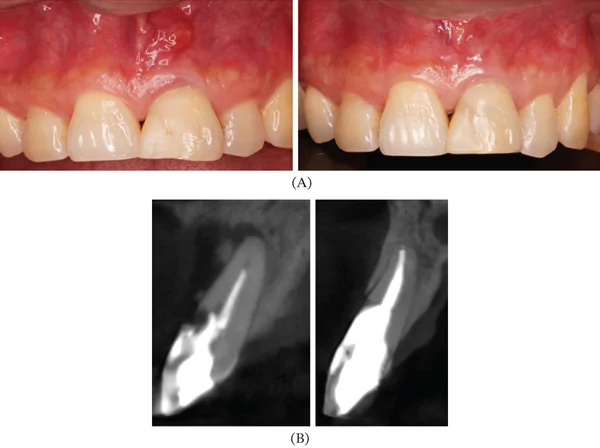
Comparison of the (A) initial and 28‐month follow‐up clinical and (B) CBCT images demonstrating lesion resolution and maintenance of periodontal and endodontic stability.
